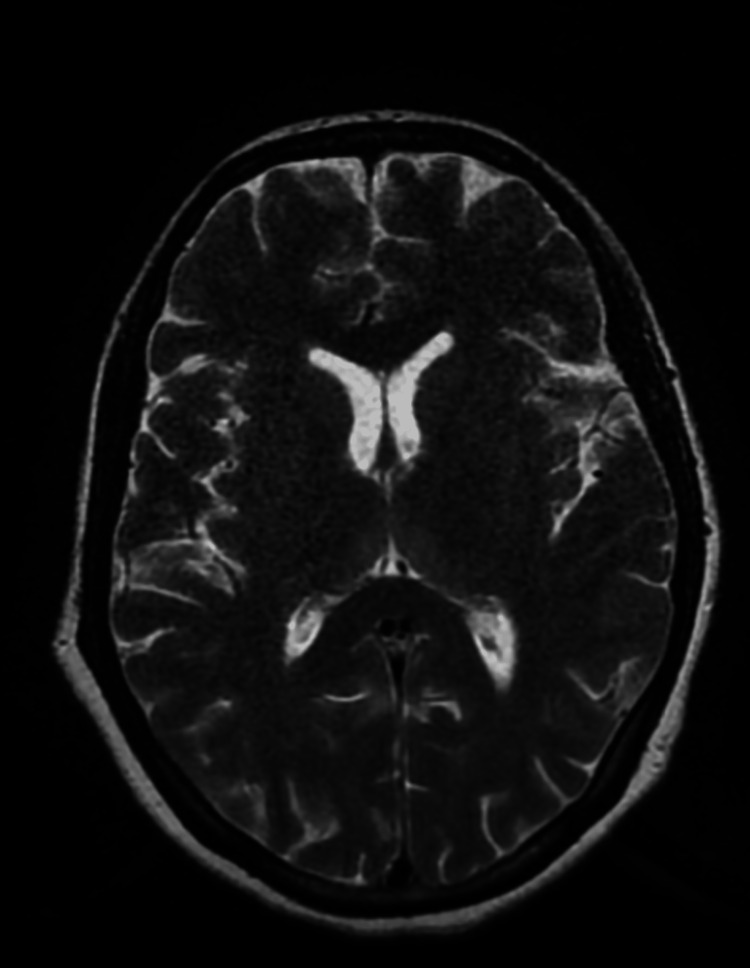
Axial T2WI of plain brain magnetic resonance imaging suggestive of no obvious abnormalities T2WI, T2-weighted image
